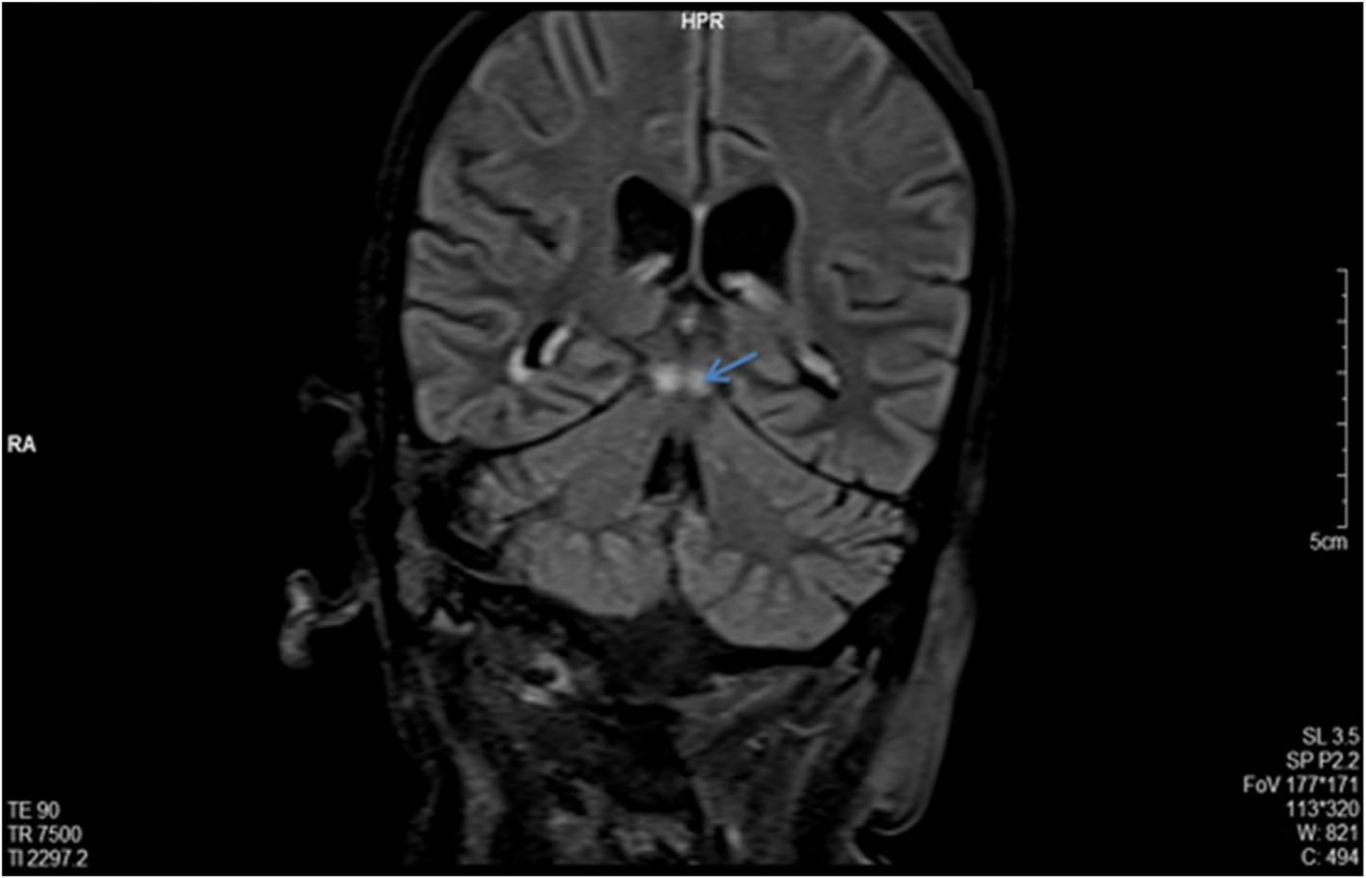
Coronal section of Cerebral MRI in FLAIR sequence showing: Hyper signal of the mammary bodies
